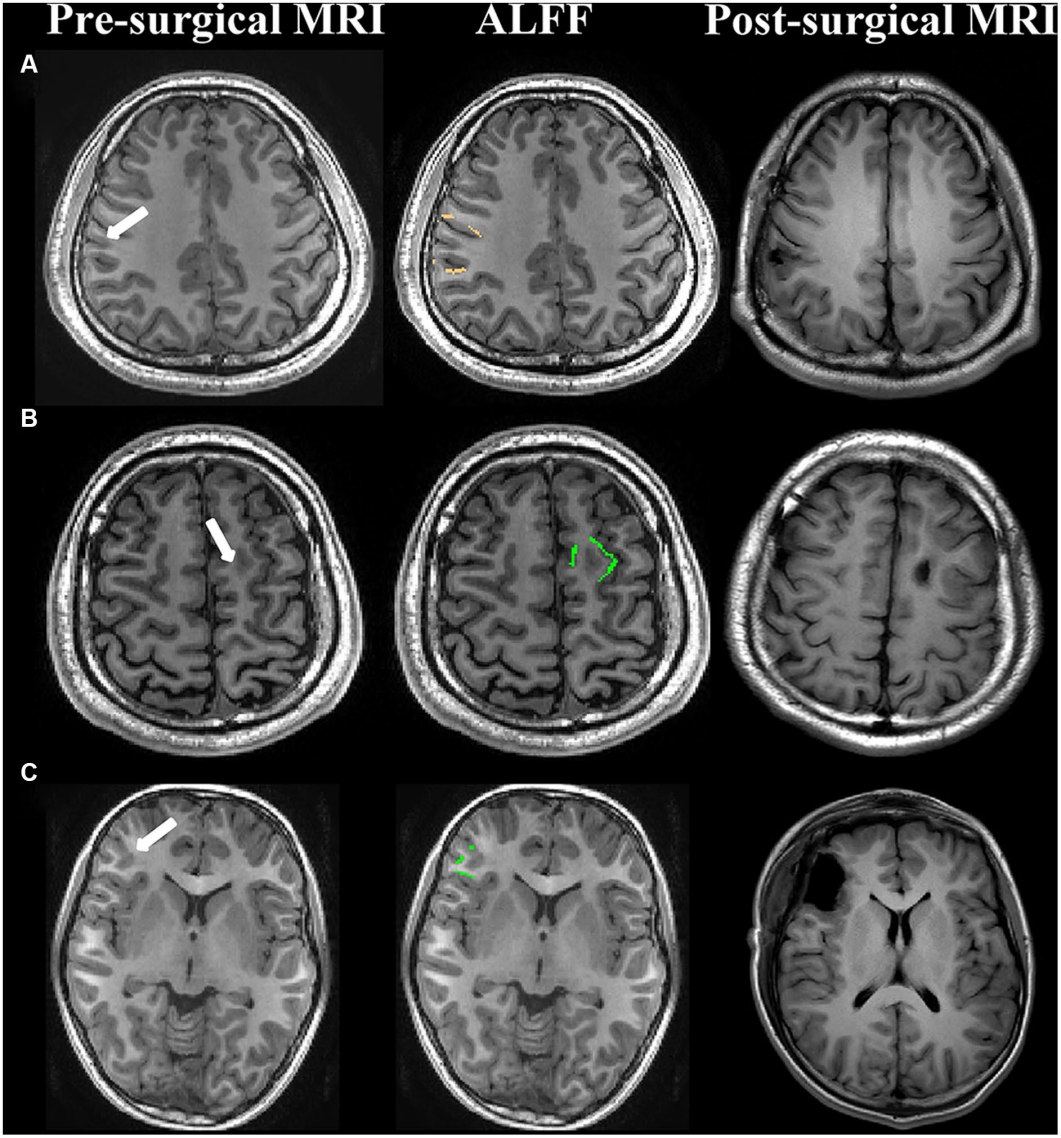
Examples of patients with abnormal ALFF maps within or outside the surgical regions. (A) FCD type IIa (seizure-free); (B) FCD type IIb (seizure-free); (C) FCD type IIb (seizure-free); First column: presurgical 3-dimensional T1-weighted images. The white arrow represented the lesion. Second column: the abnormal amplitude of low-frequency fluctuations maps in FCD type IIa and FCD type IIb were ipsilateral perilesional GM layer 6 (copper) and layer 2 (green), respectively. Third column: post-surgical MRI indicating the site and the extent of resection.
